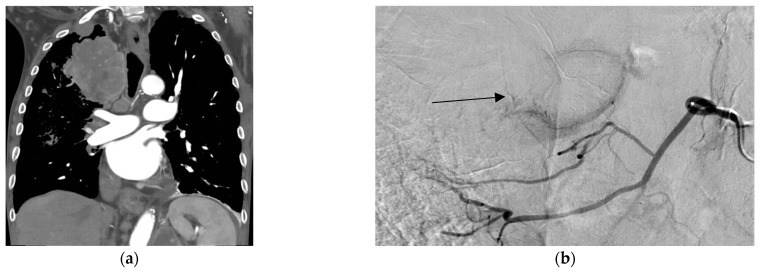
CT and selective angiography of right apical lung tumor in a patient with hemoptysis. A 63-year-old male presented with hemoptysis from a right upper lobe (RUL) mass. (a) Coronal CT of the chest shows a large necrotic right suprahilar mass. (b) Selective right bronchial arteriogram demonstrates tumor blush (arrow).
